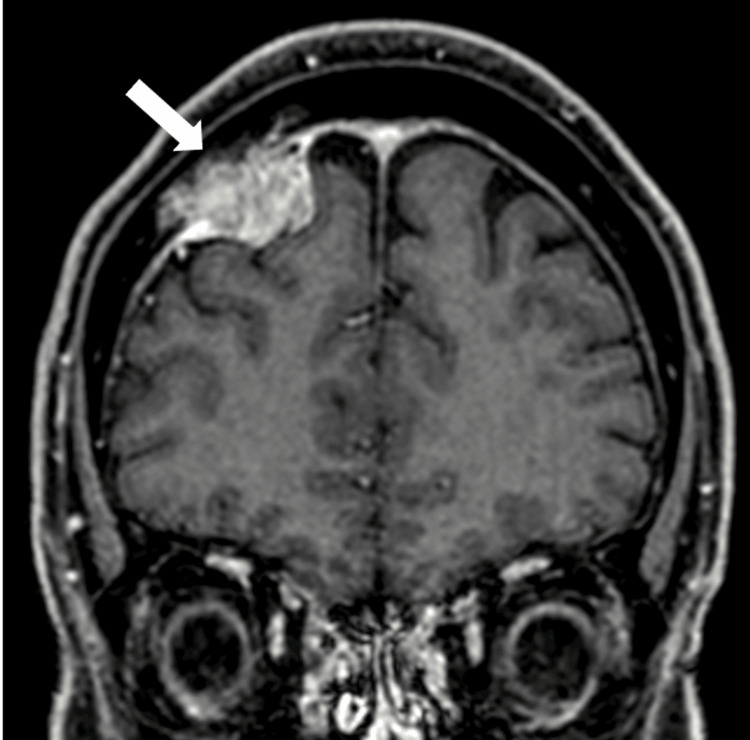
Preoperative head contrast MRI revealing a meningioma with tumor invasion into the diploic layer in the right frontal skull bone (white arrow)
